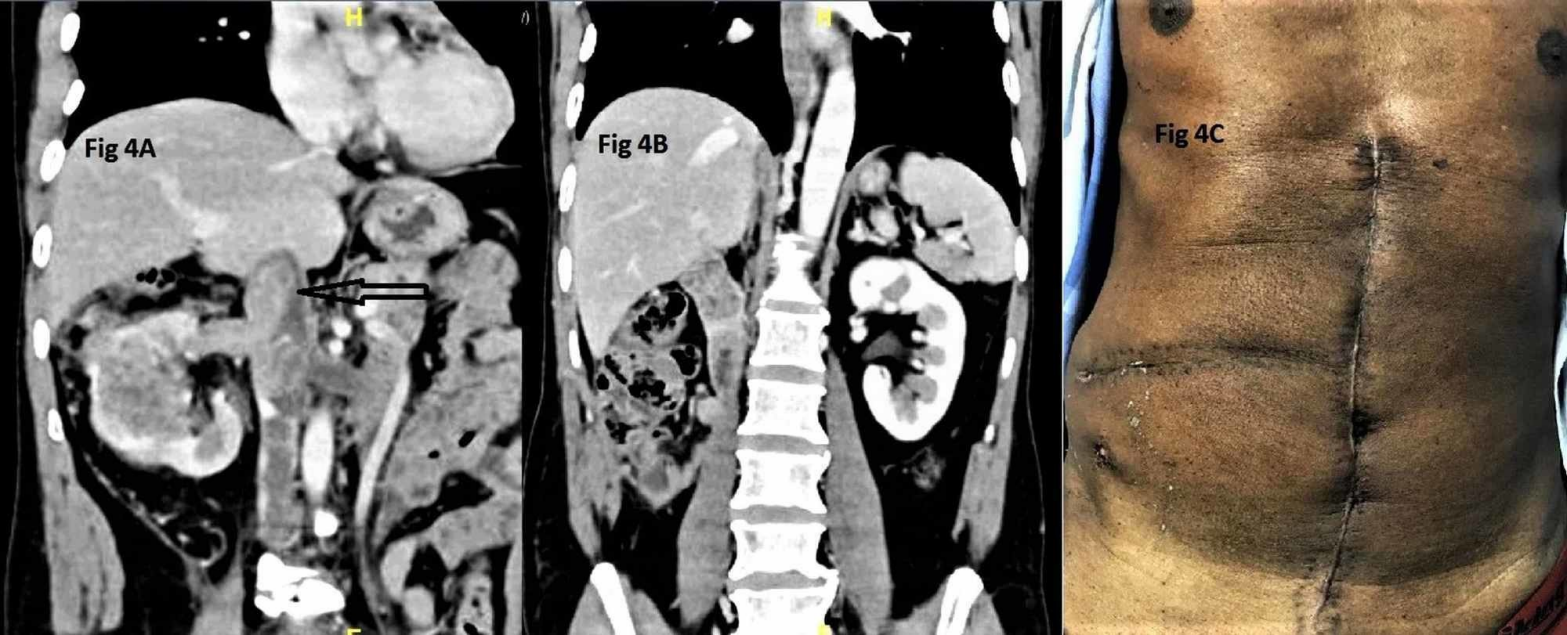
A: Preoperative contrast enhanced CT showing mass in right kidney with arrow indicating enhancing tumor thrombus in inferior vena cava and long bland thrombus in infra renal vena cava. B: Three months postoperative CT showing no evidence of recurrence. C: Healthy double Makuuchi scar after three months of surgery.
